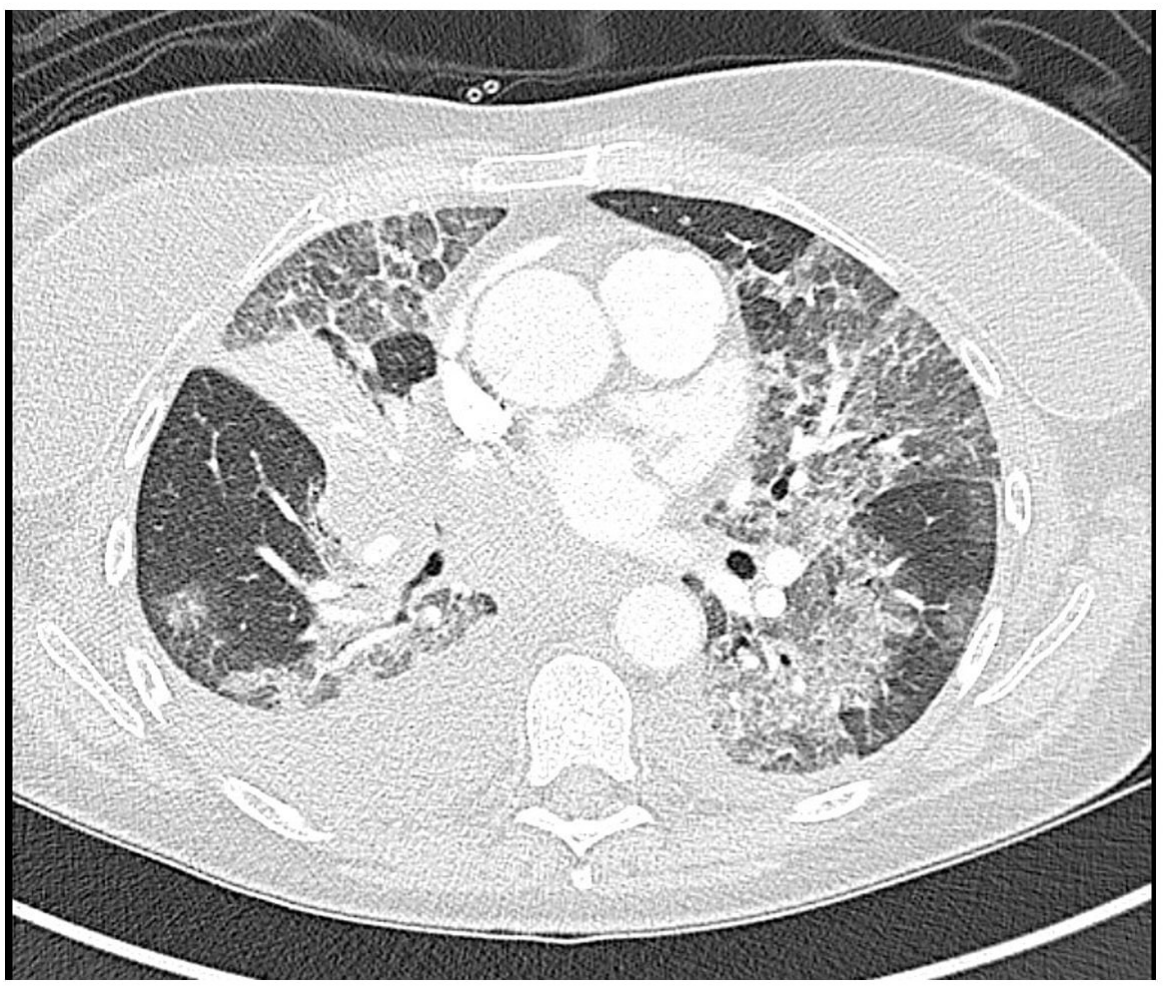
CT angiogram of the chest 38 h after initiation of brigatinib showing extensive bilateral ground-glass and reticular opacities along with interlobular septal thickening. There is also bilateral lower lobe atelectasis, new complete right middle lung atelectasis, and new right pleural effusion.
